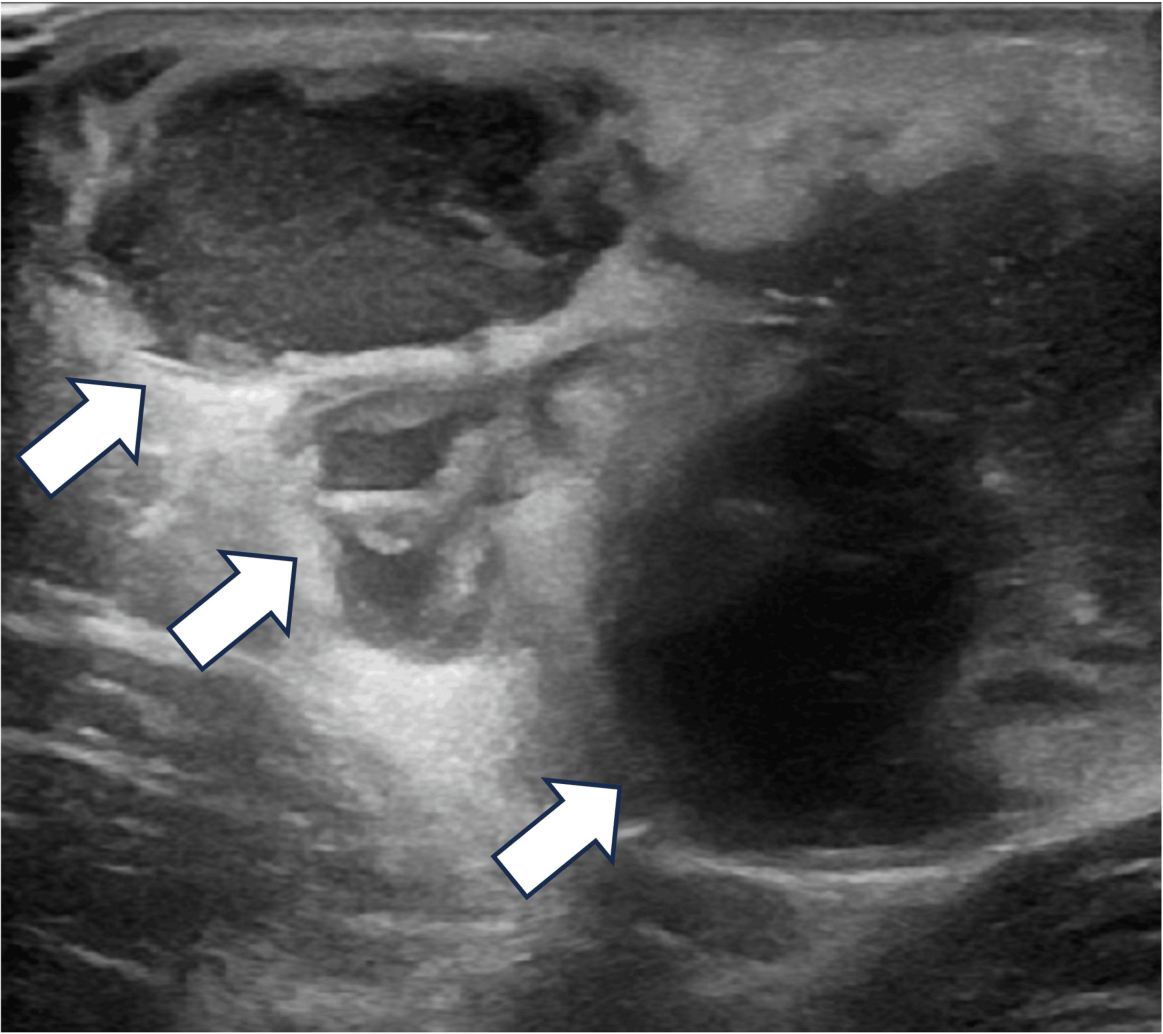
Pre-drainage ultrasonography of the right thigh. Ultrasonography of the anterior right thigh demonstrating a well-defined hypoechoic lesion with internal septations, consistent with an abscess. Surrounding soft-tissue echogenicity suggests inflammatory changes.
